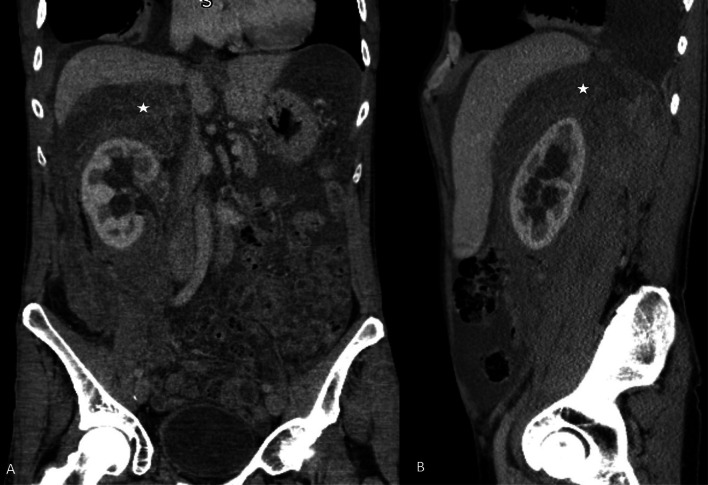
67-year-old male with biphasic type of malignant peritoneal mesothelioma. a, b CT coronal and sagittal reformatted images showing right perinephric soft tissue density mass (*), right hydronephrosis and ascites. This patient also had concurrent right intra-thoracic disease
